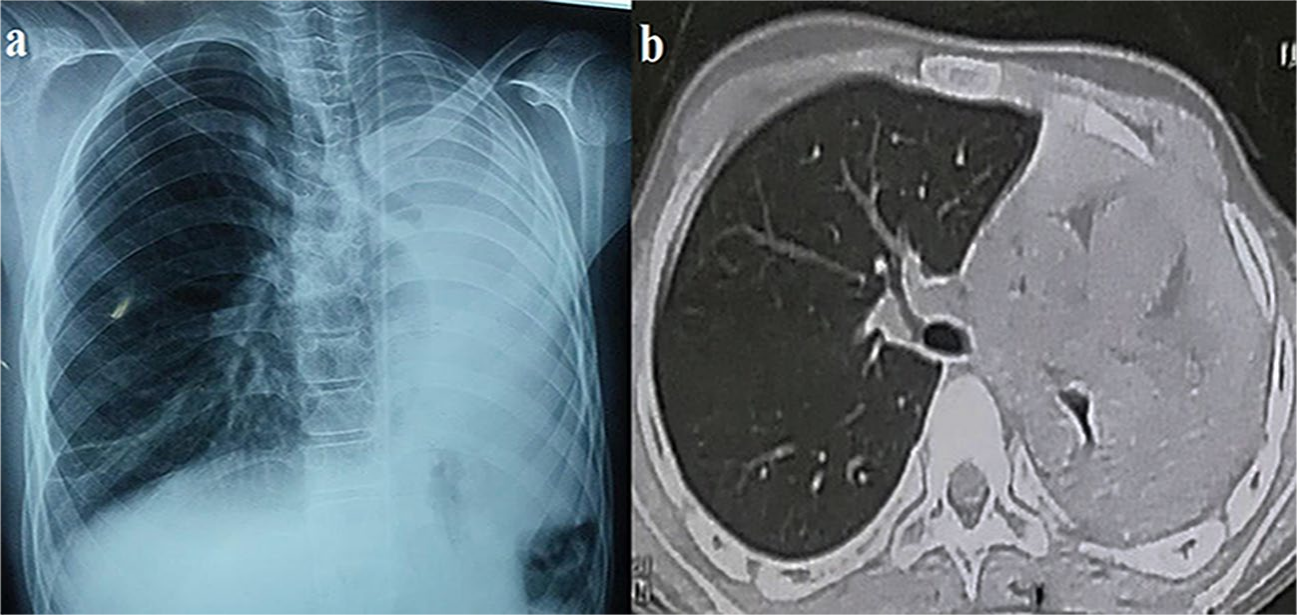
(a) Chest radiograph, and (b) CT chest showing left hemithorax opacification
